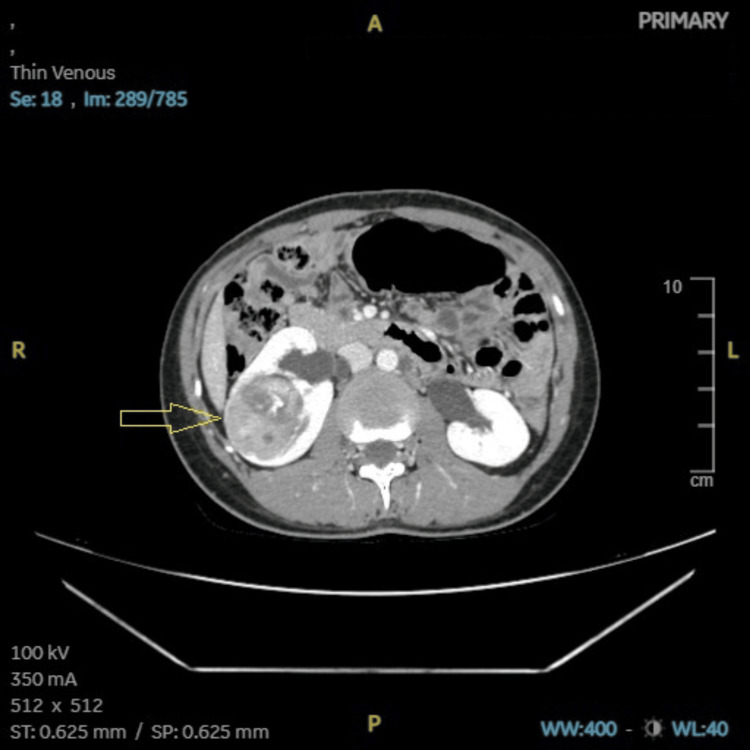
Case 3: CECT (abdomen and pelvis), right renal mass measuring 4.4 × 3.6 × 5 cm (arrow). CECT: contrast-enhanced computed tomography, A: anterior, R: right, L: left, P: posterior, ST: soft tissue, SP: soft tissue window (preset), WW: window width, WL: window level.
